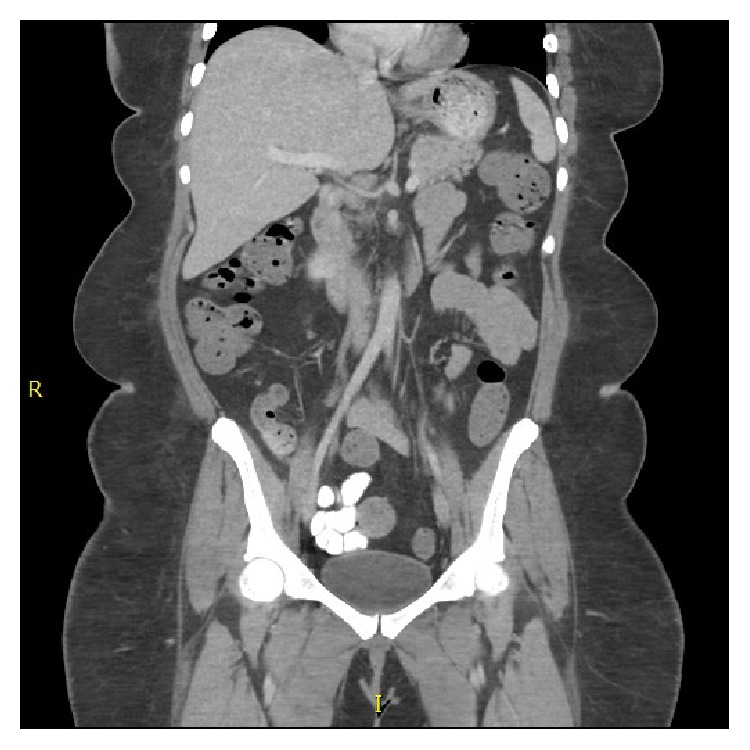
Showing resolution of Pneumatosis Intestinalis on CT imaging.
